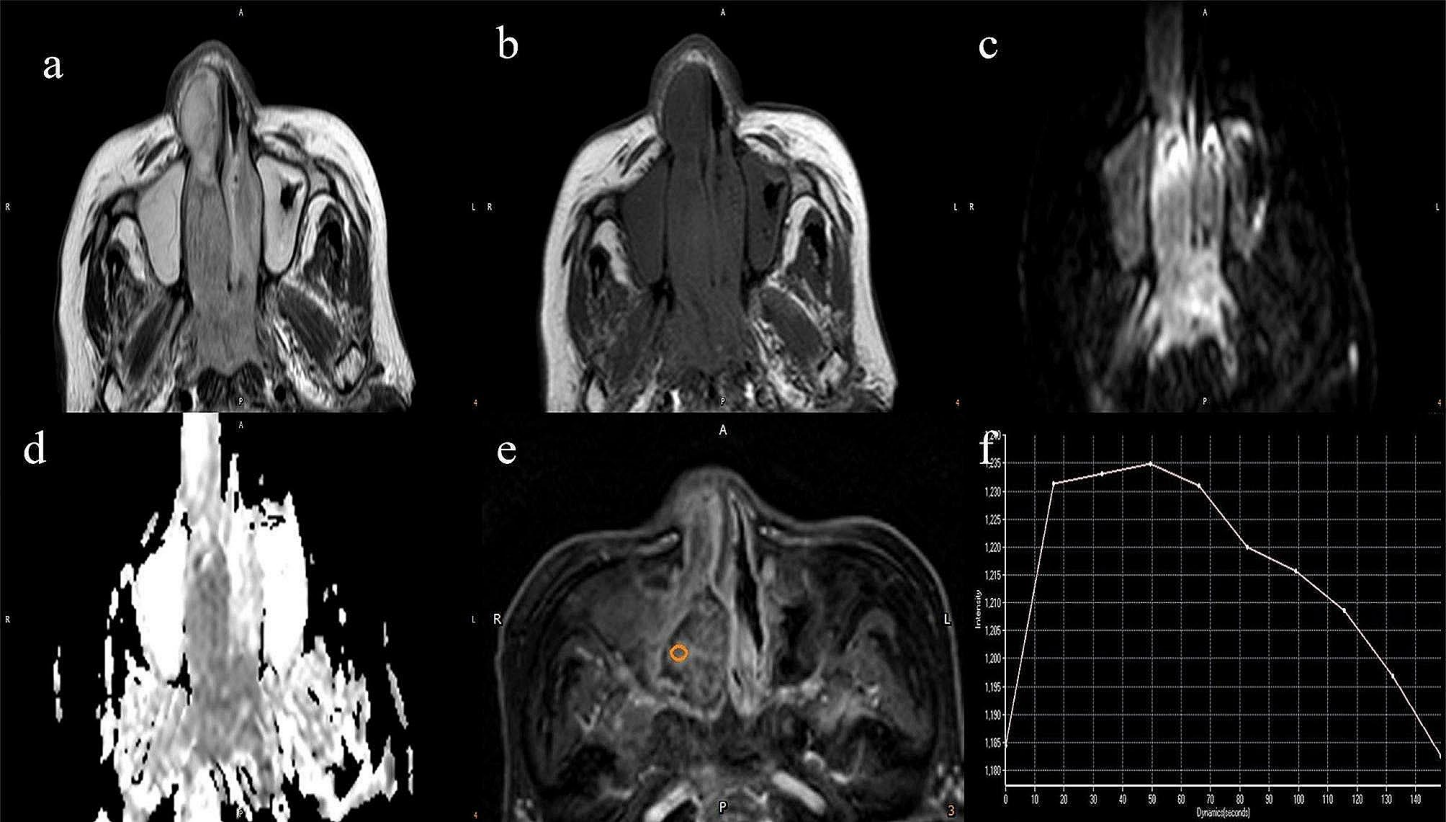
An 82-year-old female patient with sinonasal carcinoma, demonstrated heterogenous signals in the right nasal cavity, nasal cavity, nasopharyngeal cavity, and maxillary sinus with slightly high T2WI (a) and slightly low T1WI (b). The DWI (c) demonstrates high signal intensity, while the ADC map (d) shows decreased signal. The measurement method of ROI is the same as Fig. 2. Image e shows heterogeneous enhancement of lesion. Image f displays a type III TIC
